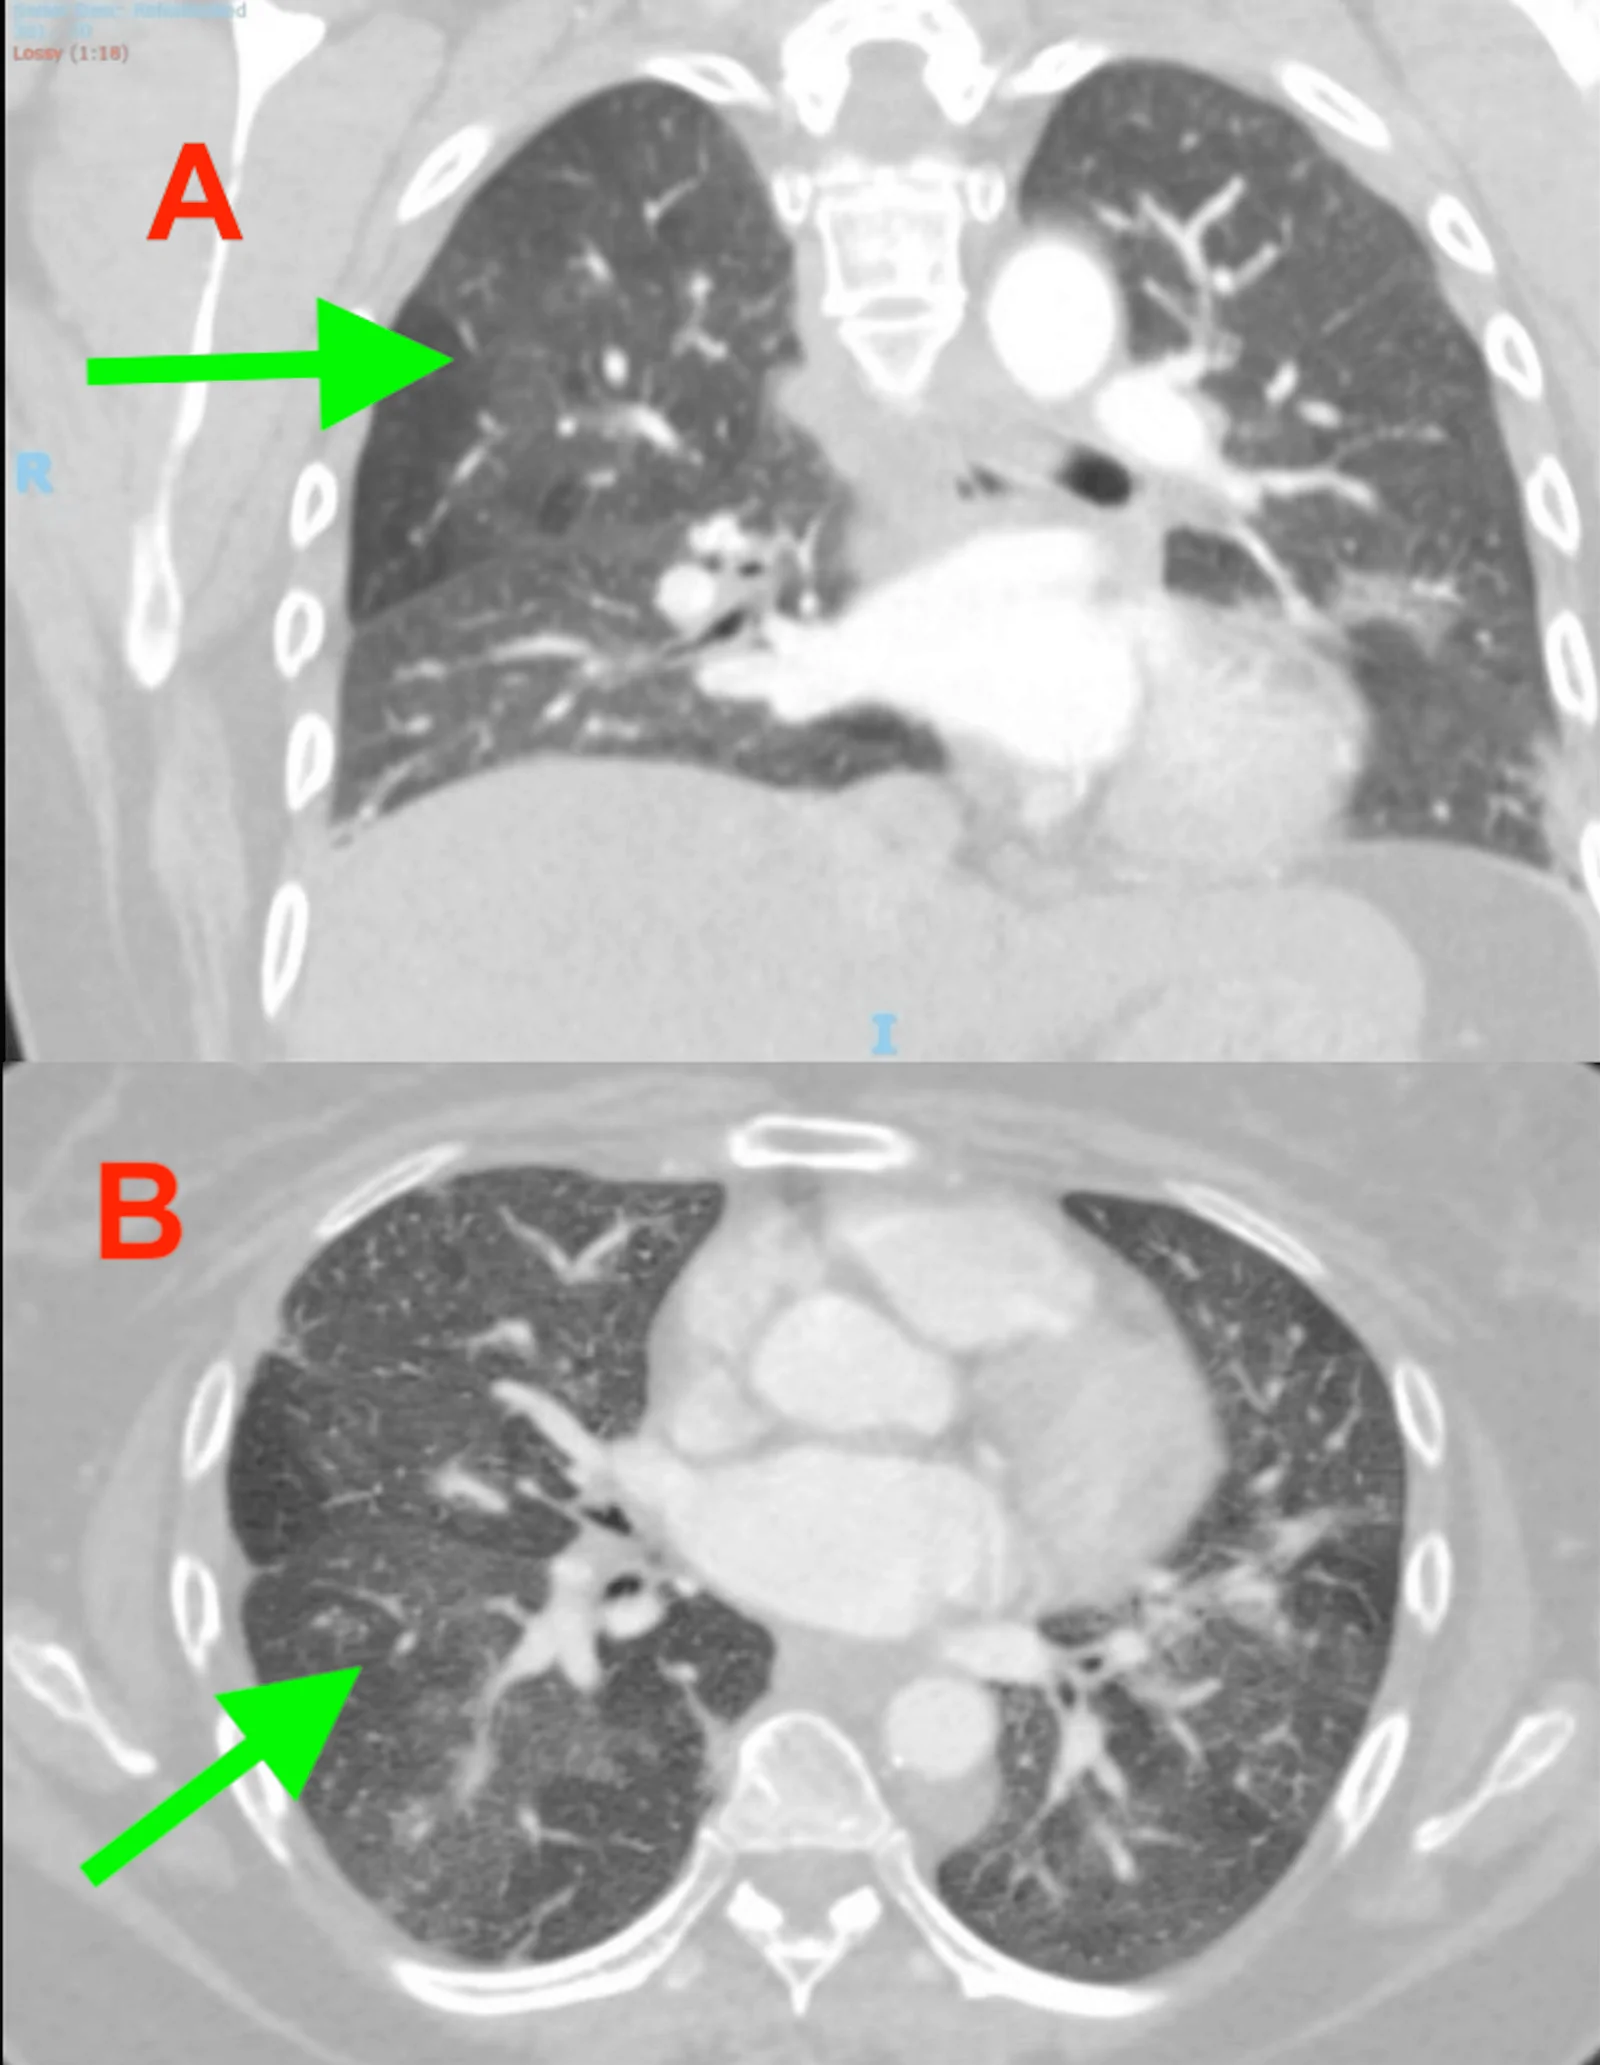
CT Chest (10/2010) - coronal view (A) and transverse view (B) showing bilateral ground-glass opacification of the lung fields (green arrows)
